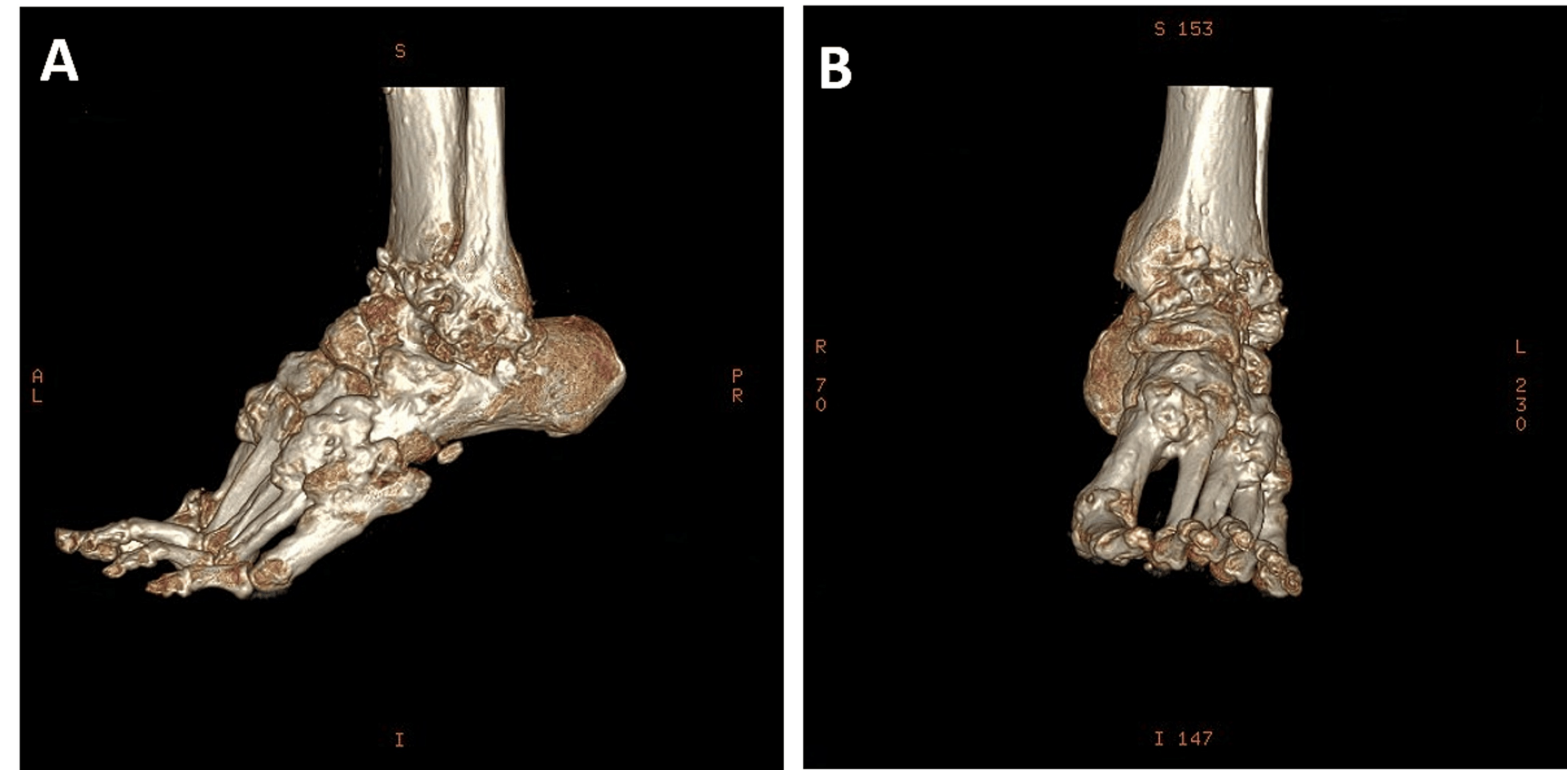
Preoperative three-dimensional computed tomography scan of the left ankle. The images show (A) lateral view and (B) anteroposterior view. Multiple bony spurs are evident around the ankle joint, particularly in the anteromedial to the anterolateral regions.
